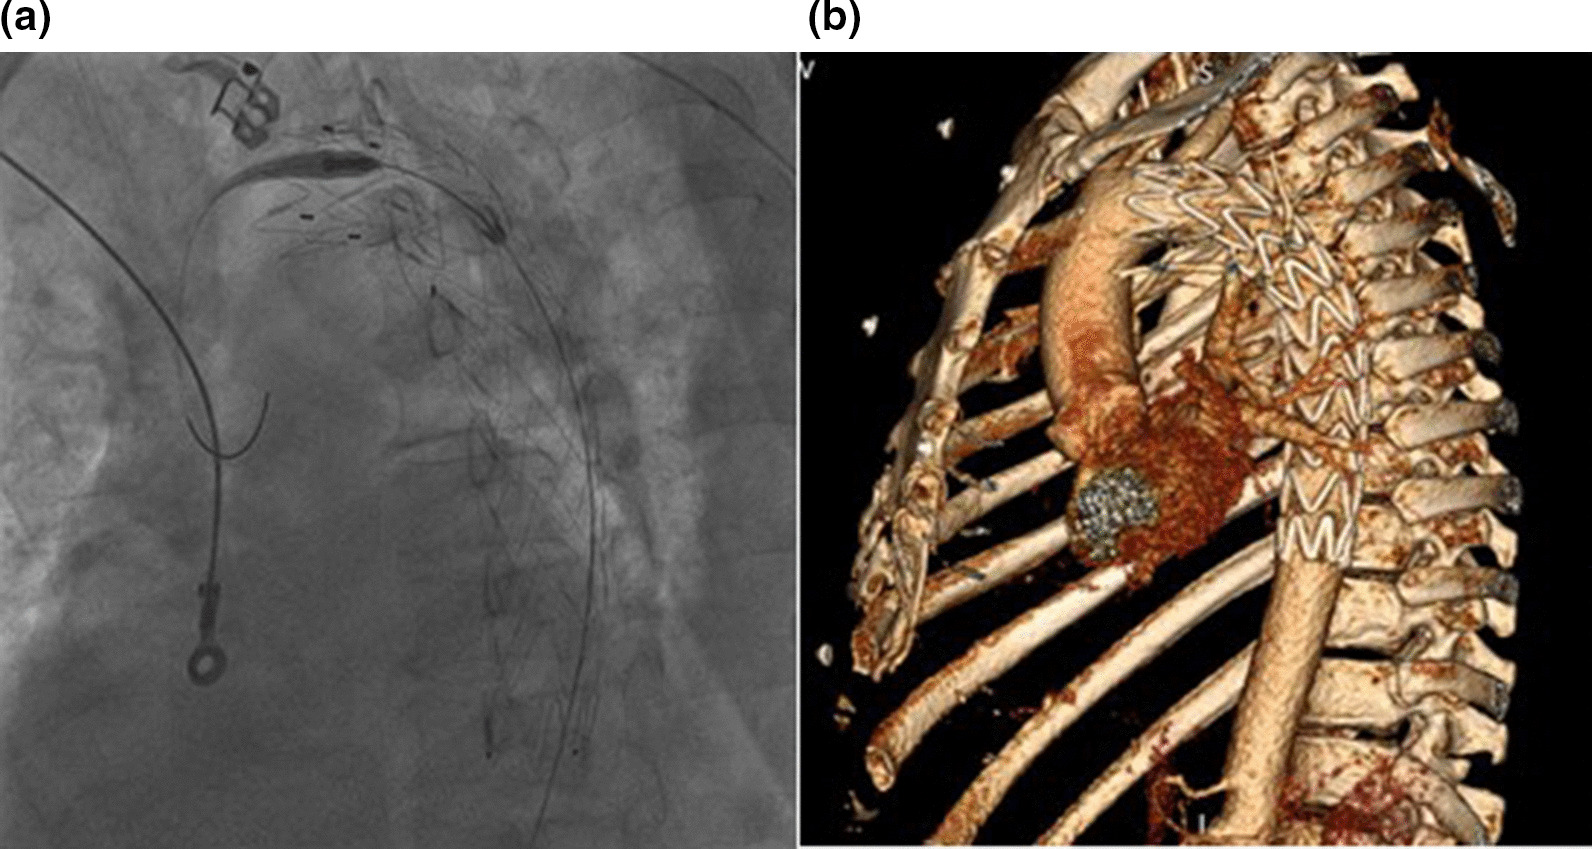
Angiography (a) and CT scan reconstruction (b), showing a normal position of the aortic stent graft
